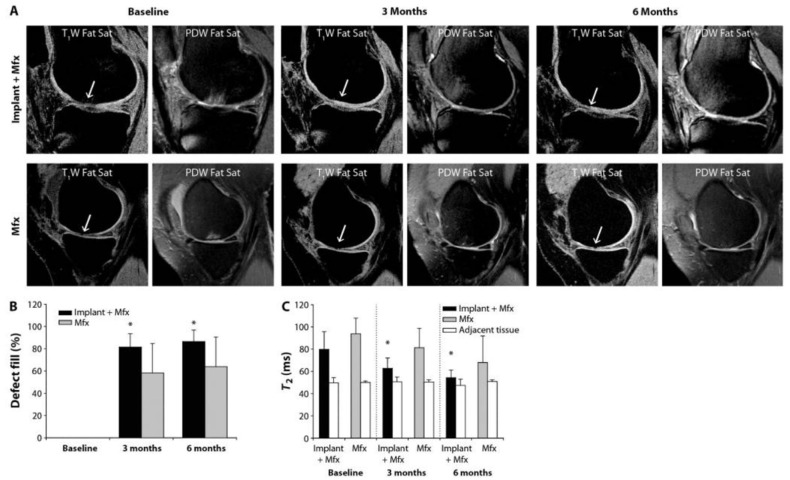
Imaging from a clinical evaluation of cartilage repair. (A) Magnetic resonance imaging of cartilage repair with implant and microfracture compared to microfracture alone; (B) quantification defect fill; and (C) T_2_ relaxation times. * *p* < 0.05. Adopted from [92].
